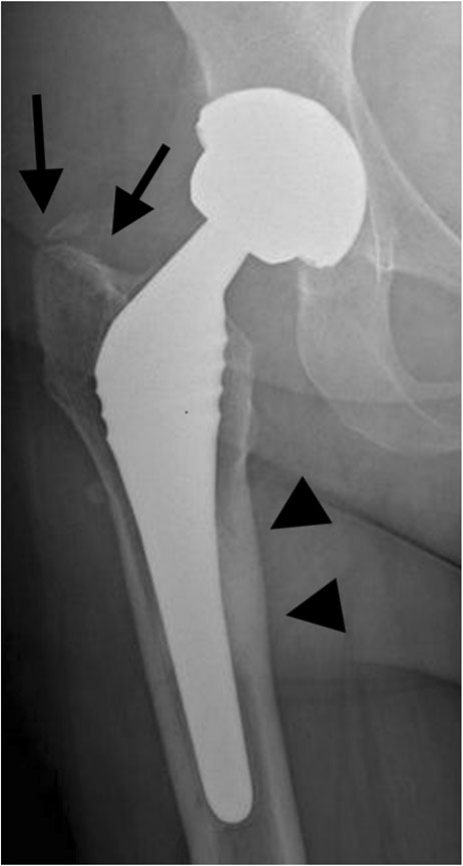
AP radiograph. Cementless total hip arthroplasty. Stress shielding. Cortical hypertrophy in Gruen zone 1 (*arrowheads*) and adaptive atrophy in Gruen zone 6 (*arrows*) as different parts of reactive bone remodelling
